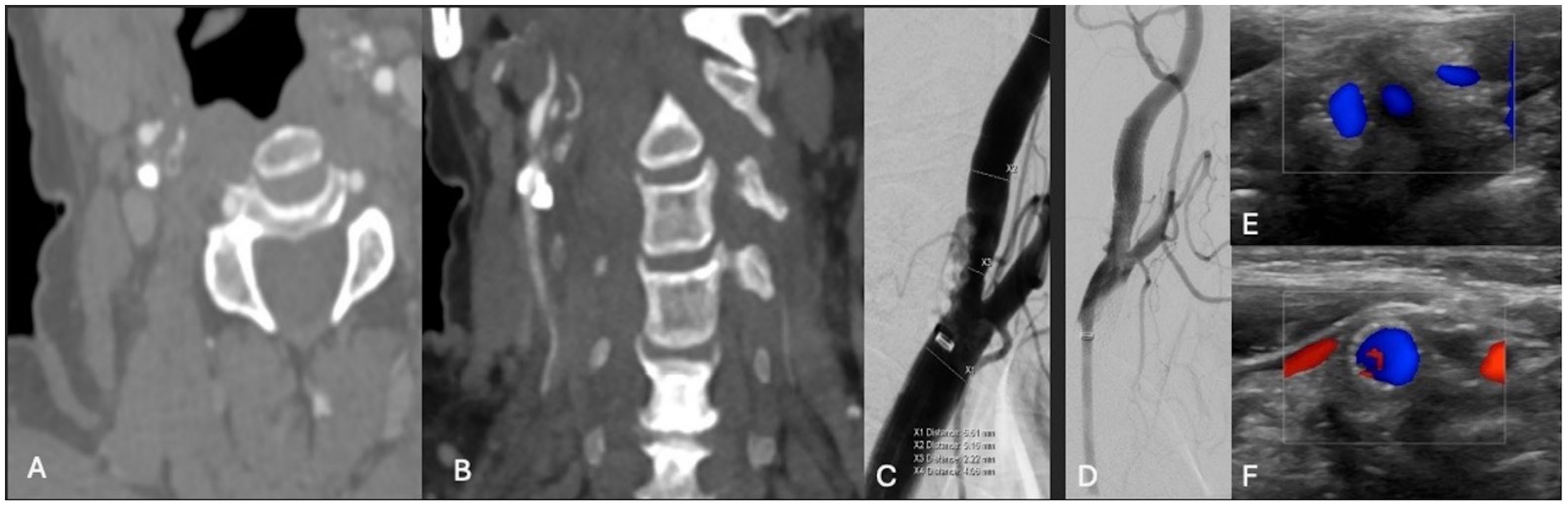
(A,B) Axial and coronal CTA demonstrating right ICA circumferential calcified stenosis. Catheter angiography demonstrating (C) severe R ICA stenosis. (D) Catheter angiography demonstrating improved caliber and flow following lithotripsy, stenting and angioplasty. (E,F) Duplex ultrasonography of the right carotid bulb and ICA following lithotripsy, stent and angioplasty demonstrating stent patency and normal peak systolic velocity.
